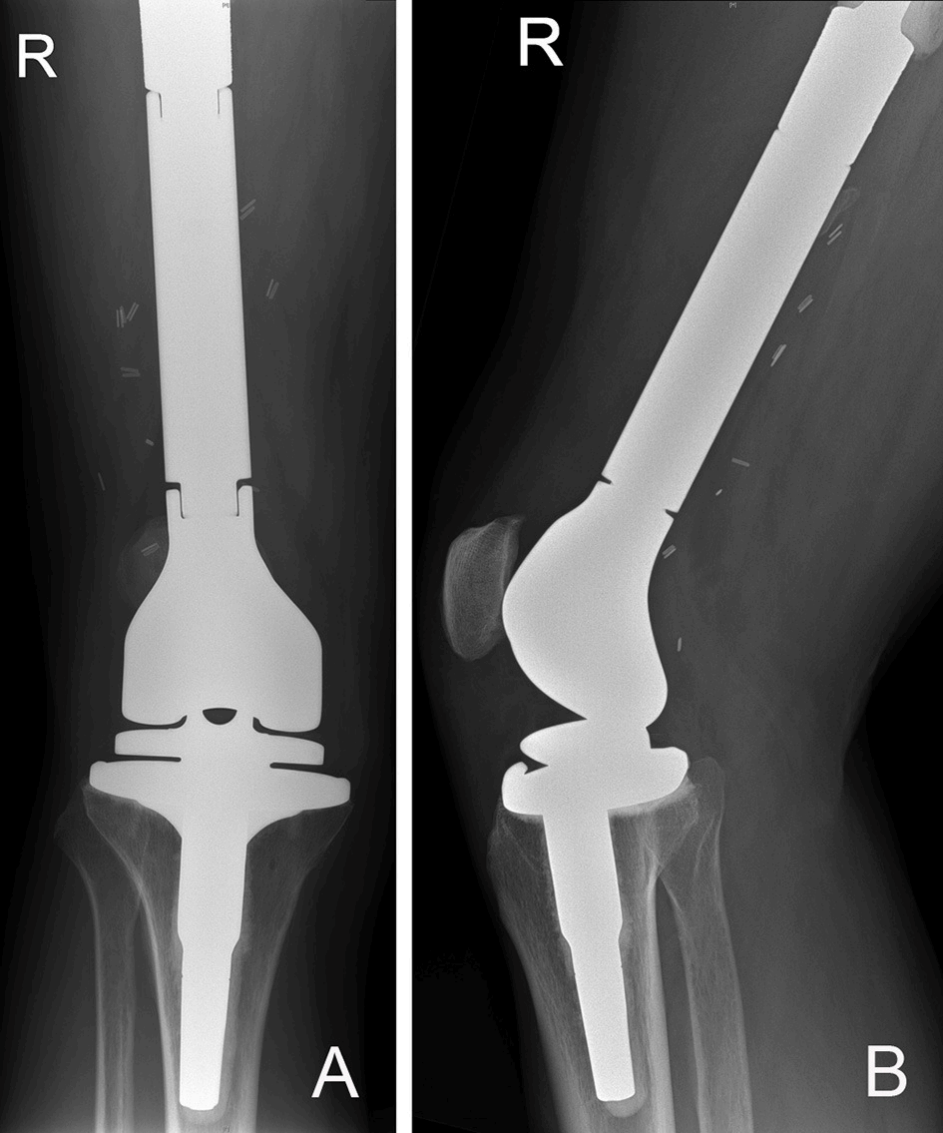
(A) AP and (B) lateral radiographs of a right distal femur modular endoprosthesis. The modularity of these implants allow for reconstruction of long segments of bone defects.
